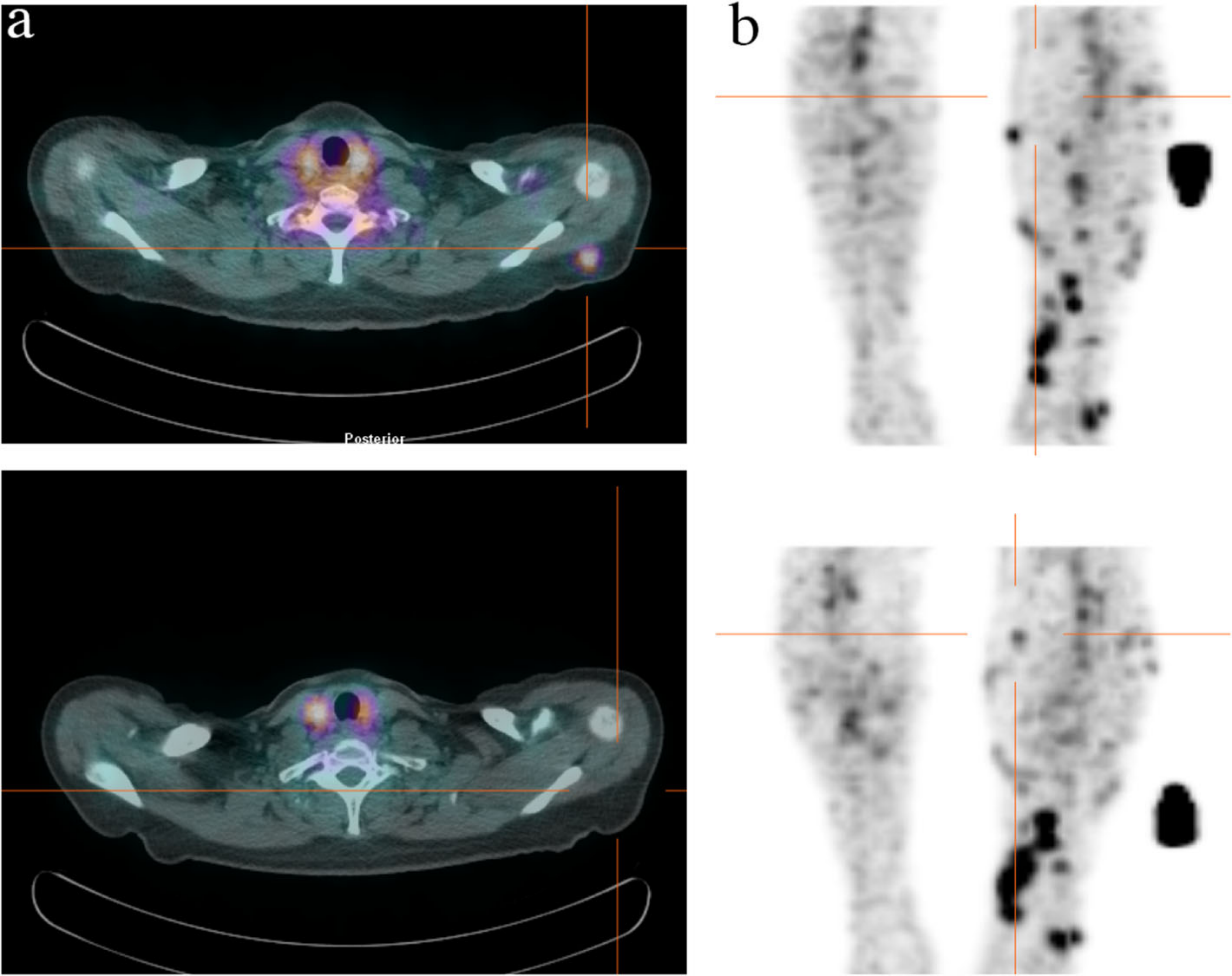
^99m^Tc-IL2 SPECT-CT in patients affected by metastatic melanoma before (top) and after (bottom) immunotherapy with ipilimumab. a) Patient with a ^99m^Tc-IL2-positive lesion that responded to therapy. b) Multimetastatic patient with different degree of uptake of ^99m^Tc-IL2
